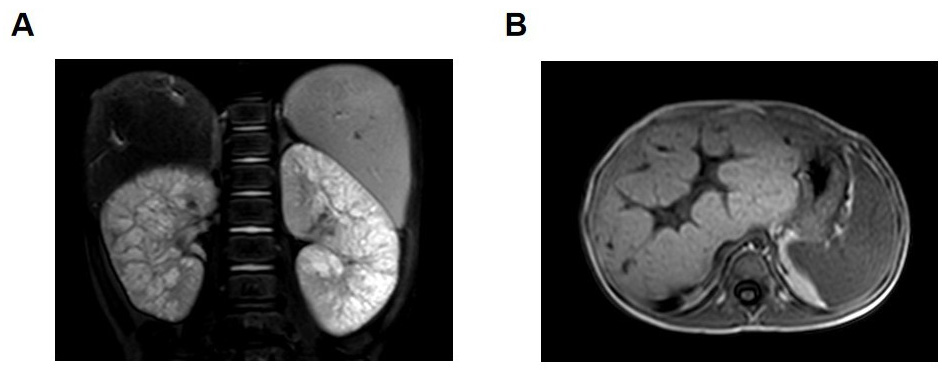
Magnetic resonance imaging (MRI) manifestations of the kidney and liver in a child with polycystic kidney disease. (A) Enlarged bilateral kidneys with multiple cysts. (B) Enlarged liver, disproportionate liver lobe ratio, and widened portal vein. MRI, magnetic resonance imaging.
